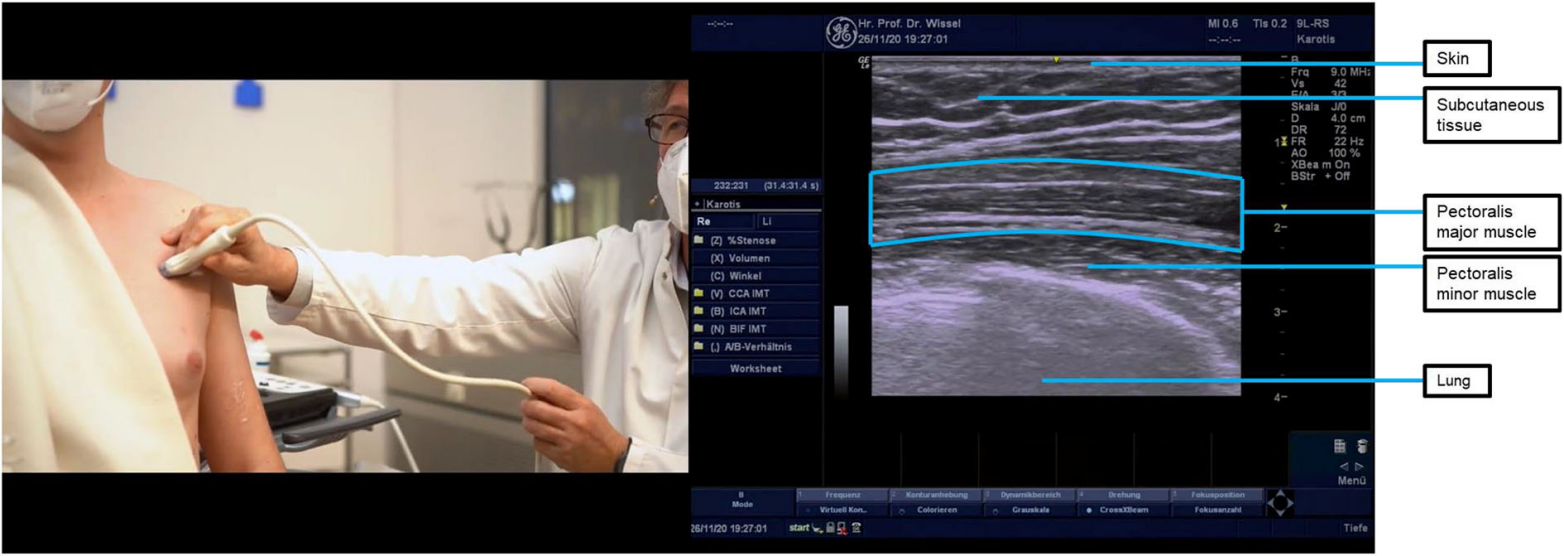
Ultrasound probe placement and image of the pectoralis major muscle. The pectoralis major muscle is shown at the top of the ultrasound image separated by white “rips” (this fibrillar pattern indicates that the probe and muscle fibers are parallel to each other), and the pleura is shown at the bottom. Asking your patient to breathe in and out helps to identify the pleura which must be avoided when injecting. Turning the ultrasound probe through 90 degrees reveals the different fiber orientation of the pectoralis major and pectoralis minor muscles (pectoralis minor shown in the middle of the ultrasound image, which has small white dots—a starry sky appearance—indicating that the probe is somewhat perpendicular to the direction of muscle fibers.
